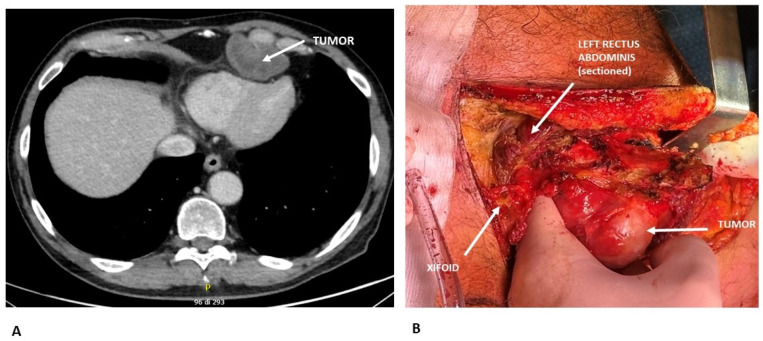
(A) Preoperative CT scan showing a chondrosarcoma arising from the 6th costo-chondral junction and compressing the cardiac surface. Radiological features suggesting chondrosarcoma: lobulated, expansive bone lesion with well-defined margins; internal low attenuation due to high water content of the cartilaginous matrix; soft tissue extension beyond the bone. (B) Intraoperative view demonstrating surgical resection of the chondrosarcoma.
